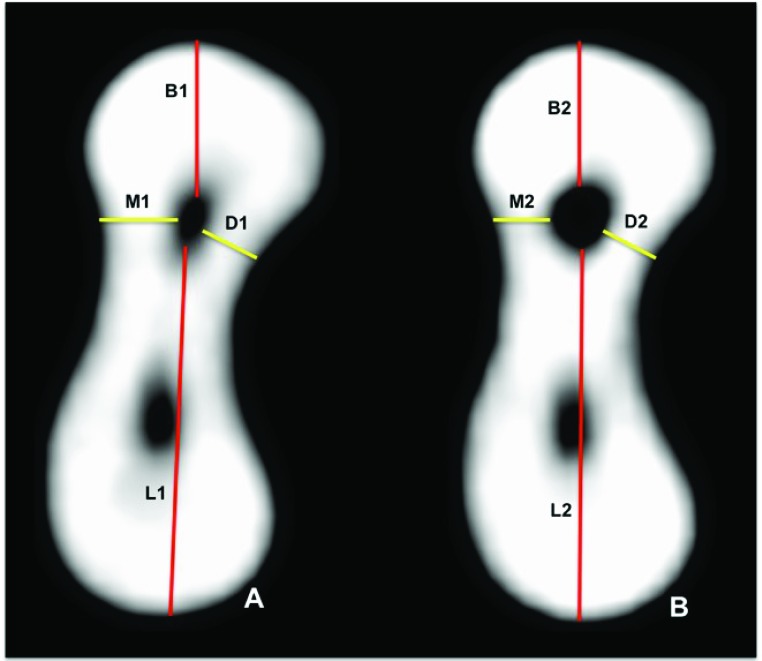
Cone-bean computed tomography scan (axial view) of mesial root of mandibular molar at 3 mm short of apex, point for measurement of mesiodistal and buccolingual distances to determine root canal transportation: (A) before root canal preparation; (B) after root canal preparation.
